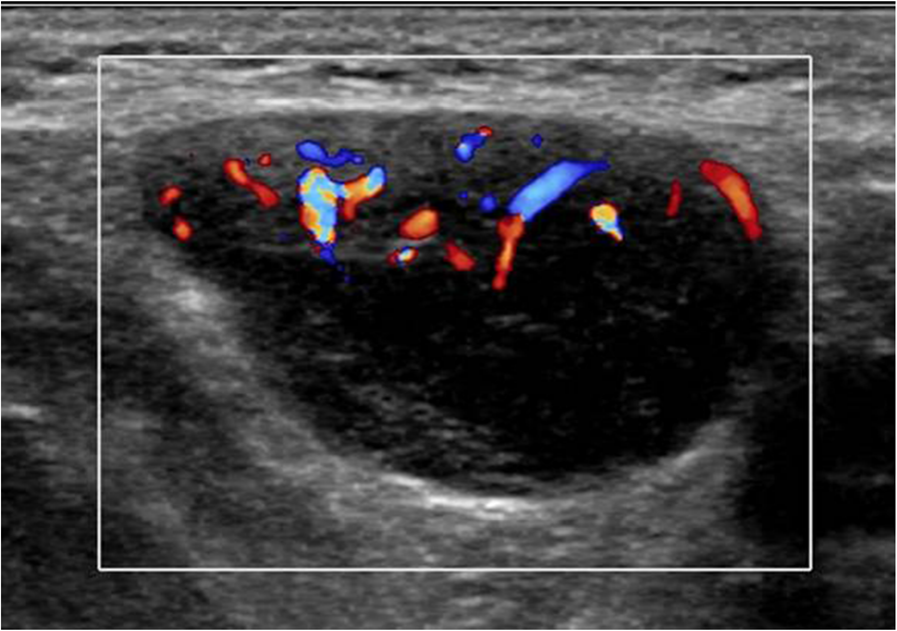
Color Doppler sonogram shows peripheral vascular flow within an ovoid hypoechoic axillary lymph node.
